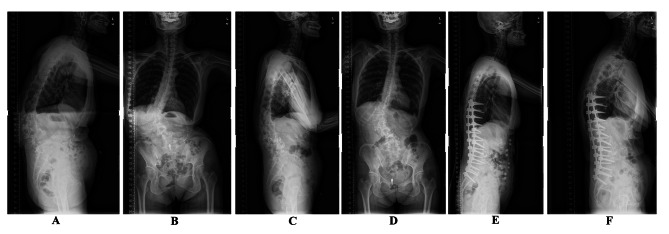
A 64-year-old female patient with low back pain treated with lateral lumbar interbody fusion combine two-stage posterior screw fixation. (A, B) A preoperative standing anteroposterior and lateral full-length spine X-ray showed the following findings: Cobb angle of lumbar curve = 58.9°, LL = 4.3°, PT = 31.3°, LL-PI = 35.6°, SVA = 12.0 cm, coronal balance was 9.2 cm. (C, D) A standing anteroposterior and lateral full-length spine radiograph at postoperatively following ALIF from L2-5. The radiograph revealed Cobb angle of main curve = 48.7°, LL = 11.8°, PT = 21.6°, LL-PI = 24.3°, SVA = 5.9 cm, coronal balance was 8.5 cm. (E) After second-stage posterior fixation from T9-S2 combined with PSF, the standing anteroposterior and lateral full-length spine radiographs showed the Cobb angle of main curve = 23.2°, LL = 35.4°, PT = 9.6°, LL-PI = 8.5°, SVA = 3.8 cm, coronal balance was 1.2 cm. (F) At final follow-up the standing anteroposterior and lateral full-length radiographs showed the Cobb angle of main curve = 24.6°, LL = 32.9°, PT = 10.9°, LL-PI = 9.5°, SVA = 4.8 cm
